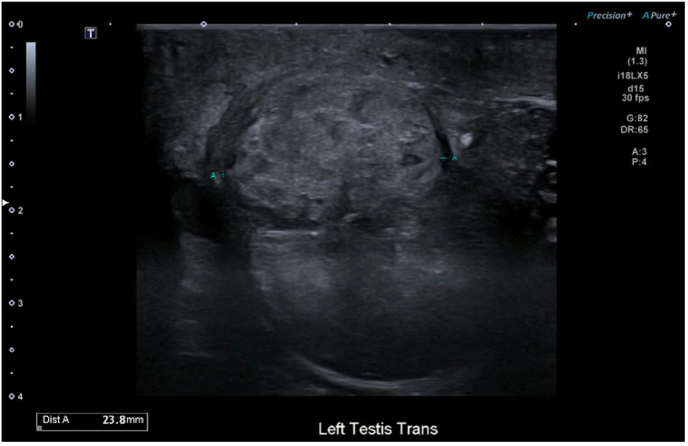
Transverse ultrasound of left testicle.
